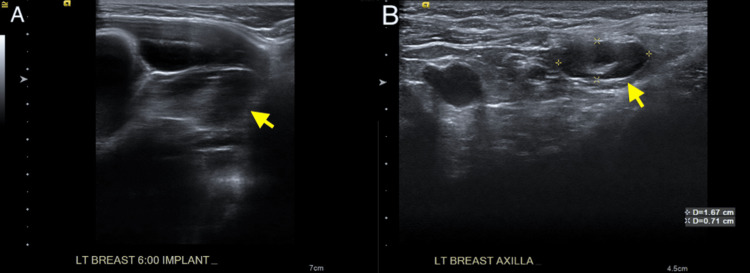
(A) Static grayscale ultrasound image of the left breast 6:00 demonstrating a complex fluid collection with internal septations surrounding the partially imaged silicone implant. (B) Static grayscale ultrasound image of the left axilla demonstrating two morphologically abnormal-appearing axillary lymph nodes with associated cortical thickening and increased size.
